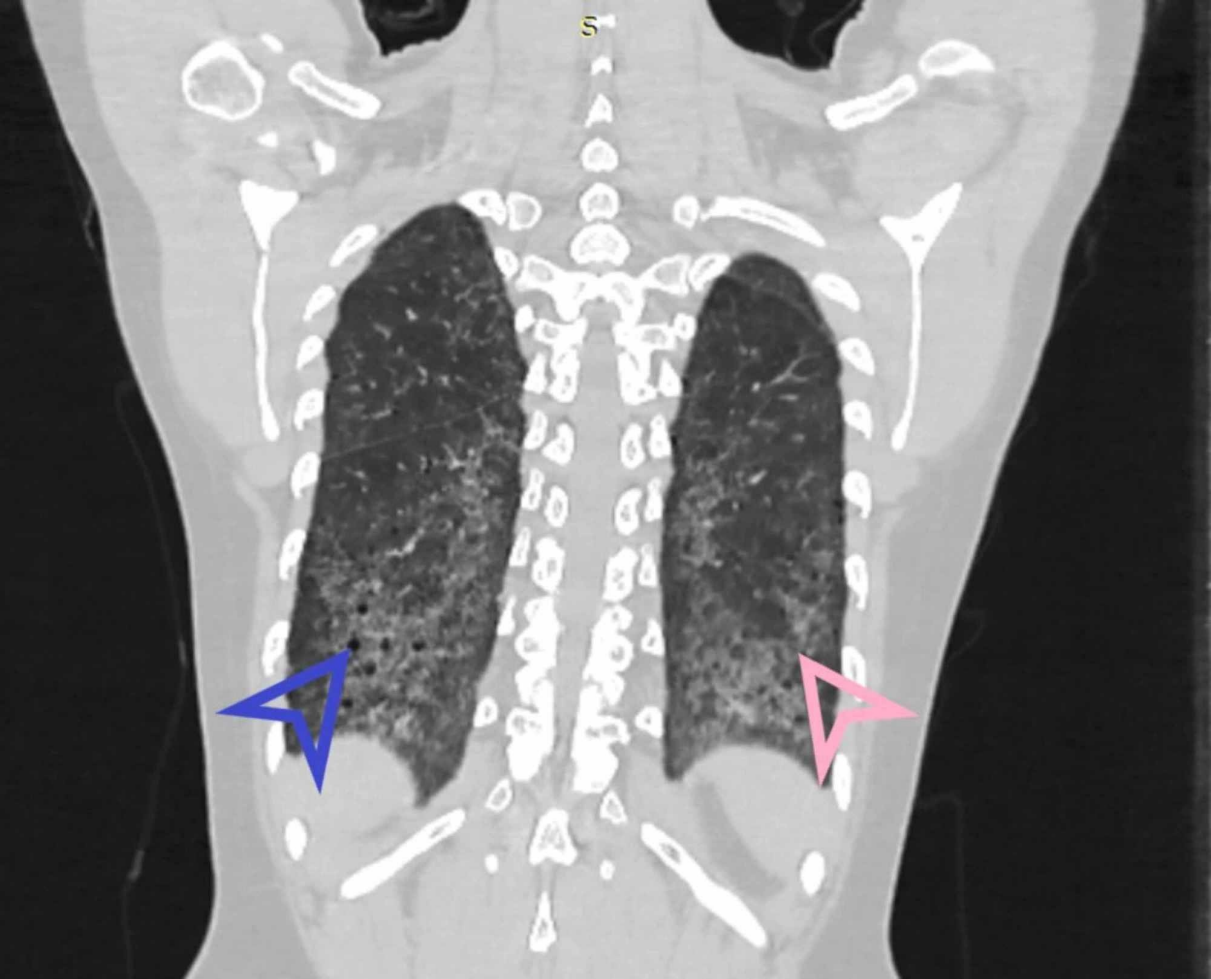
Coronal HRCT showing basal predominance of fibrosis and honeycombing in UIP HRCT - high-resolution computed tomography; UIP - usual interstitial pneumonia Blue arrowhead indicates honeycombing. Pink arrowhead shows fibrosis.
